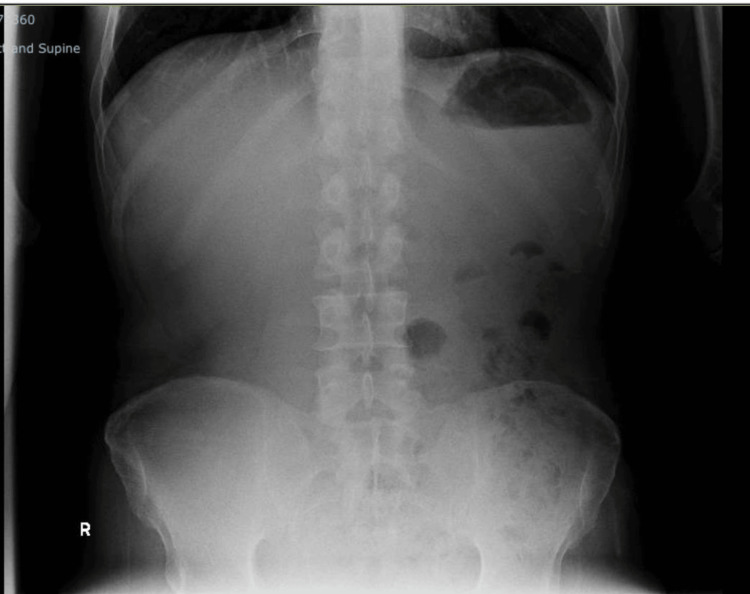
Plain abdominal radiograph Anteroposterior view demonstrating a nonspecific bowel gas pattern without evidence of obstruction.
